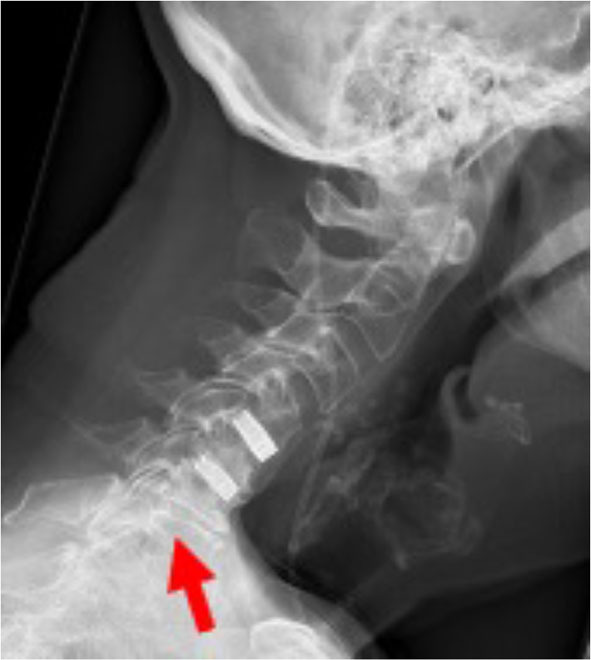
X-ray double level EIT CIF cage C4C5 and C5C6 2 years postop. Arrow indicates symptomatic level.
